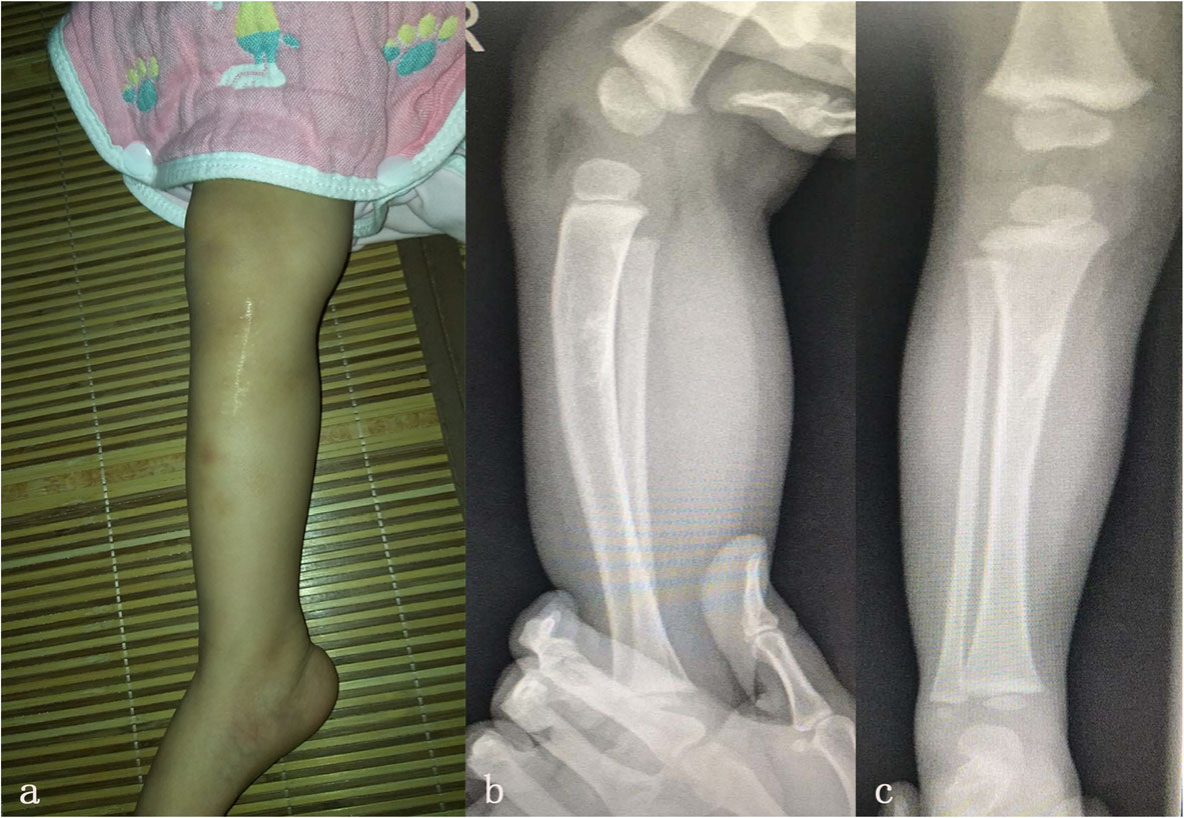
Appearance and radiographs 1 year postoperative
